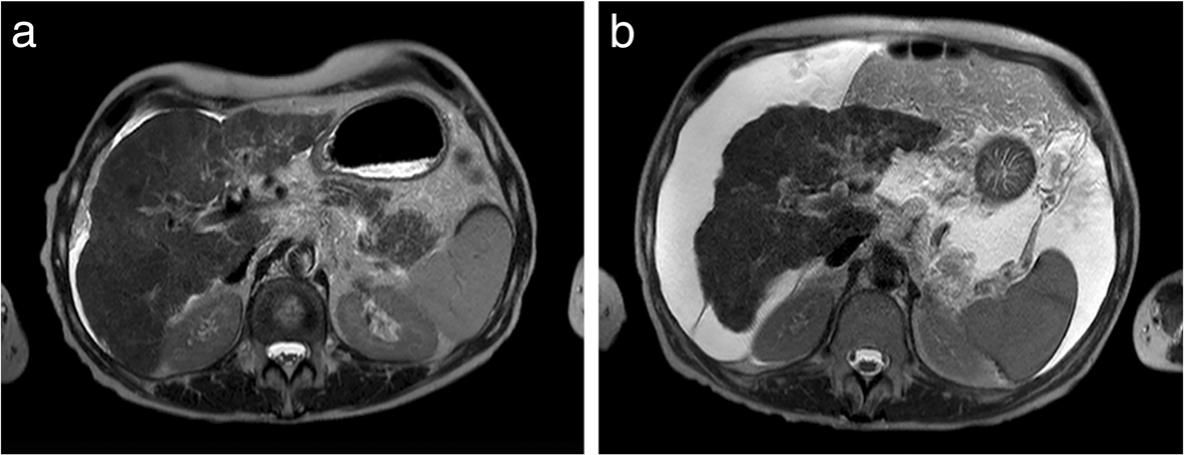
Pre- and posttreatment T2-weighted images of case 2. On the pretreatment image (a), a nodular surface of the liver is seen, consistent with cirrhosis. A small amount of ascites is present. Three months after treatment (b), the liver has atrophied impressively and the amount of ascites has substantially increased, consistent with REILD
